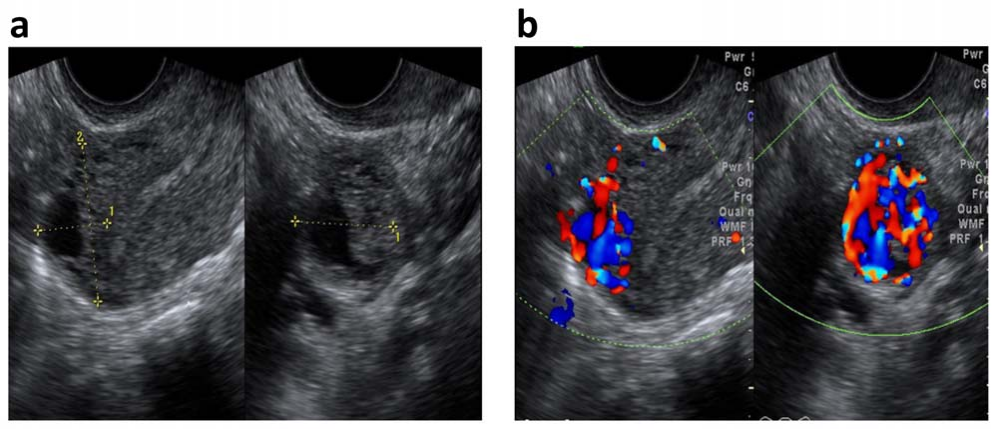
Ultrasonographic characteristics of IM. (a) On gray-scale image, the lesion was located in the uterine fundus, appearing the cystic-solid mass with unclear border (between cursors). (b) On Color Doppler image, the extremely abundant Doppler signals formed by blood flow were distributed throughout the tumor, which is named as “non-peripheral Doppler signal”. The irregular shape of cyst was the secondary arteriovernous shunts, which was fulfilled chaotic signals on color Doppler.
